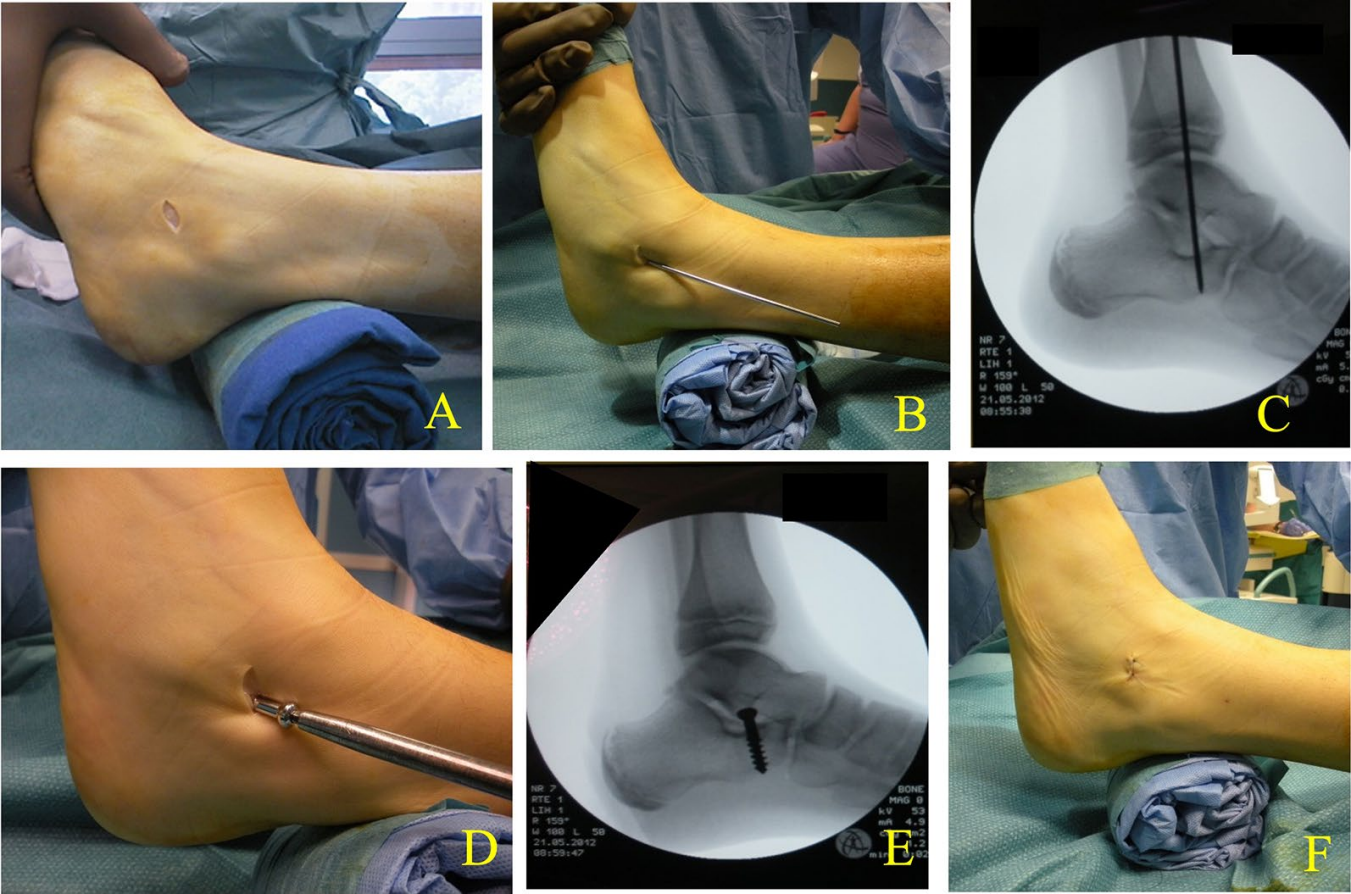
SESA step by step. A The 1 cm skin incision at the level of the sinus tarsi. B With the foot in neutral position and maximal supination, introduction of a *K*-wire into the calcaneus with an angle of approximately 20° from posterior to anterior and from lateral to medial. C x-Ray control to check the correct position of the *K*-wire. D The *K*-wire is then removed, and a 3.2 mm reamer is inserted for 2–3 mm to aid the screw entrance. The screw is then inserted until it reaches the position under the talus lateral process. Effectiveness of procedure is tested using the plantar malleoli view sign (PMVS). E Intraoperative x-ray shows correct position of the screw. F Suture in layers and skin suture
